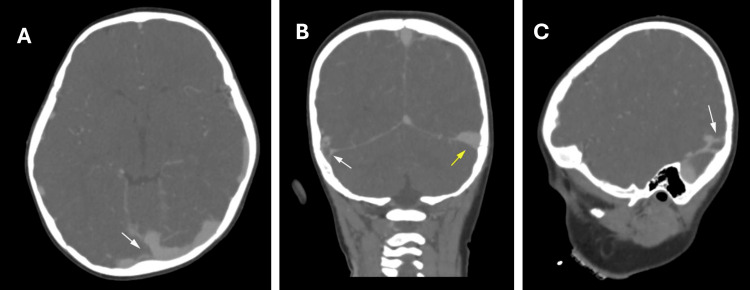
Contrast-enhanced computed tomography venography on the fourth day of admission. (A) Axial image demonstrating reduced contrast opacification of the right transverse-sigmoid sinus region compared with the left side (white arrow). (B) Coronal image demonstrating non-opacification of the right transverse sinus (white arrow) with preserved opacification of the left transverse sinus (yellow arrow), consistent with acute dural venous sinus thrombosis. (C) Sagittal reconstruction demonstrating non-opacification of the right transverse and sigmoid sinuses (white arrow).
